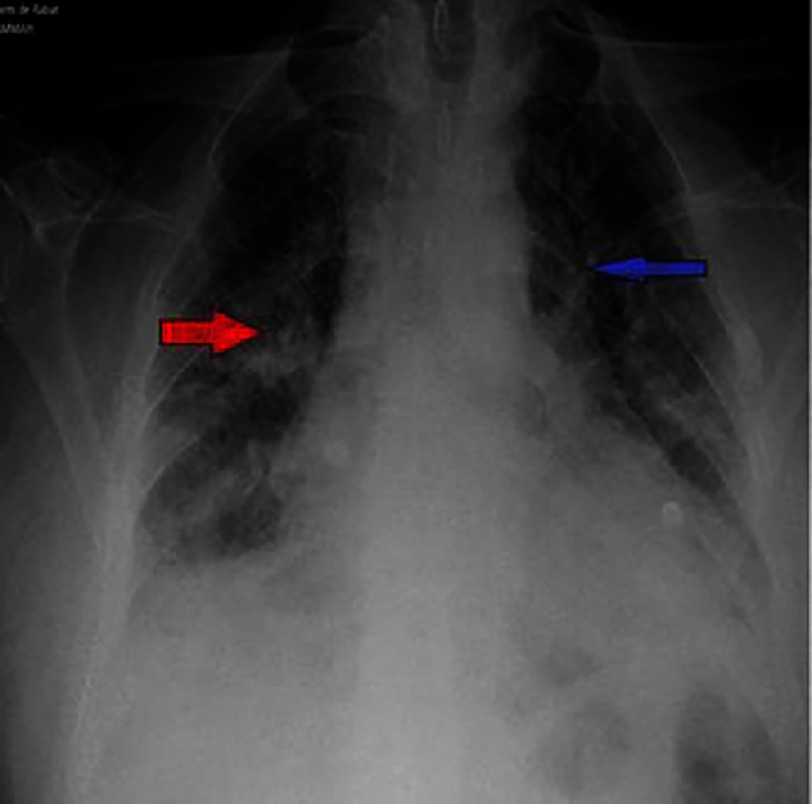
Supine posteroanterior chest X-ray showing bilateral, rounded opacities with blurred margins, predominantly in the lower two-thirds of both lung fields (red arrow), associated with linear opacities (blue arrow), suggestive of an alveolar-interstitial syndrome.
